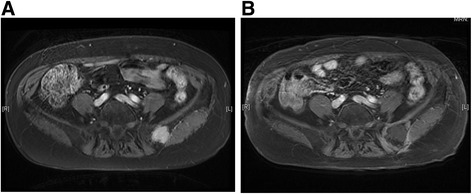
Gadolinium enhanced T1 - weighted MR image with fat suppression of an iliac metastasis from thyroid carcinoma (a) and 6 months after cryoablation (b). The effect of cryoablation is depicted as a low-signal intensity area with rim enhancement. The low-intensity area persisted during the 3 - year follow - up, a result consistent with no local recurrence
